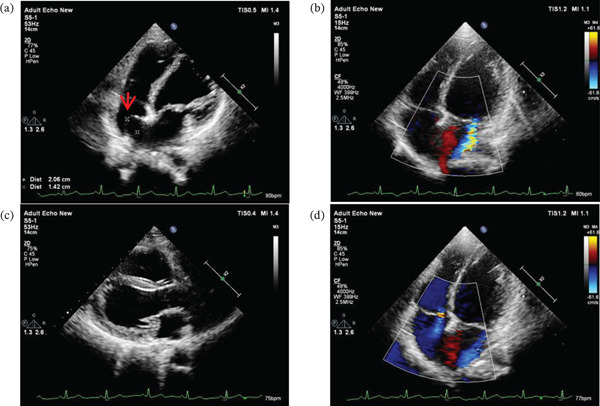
Echocardiogram of mother of proband. (a) Atrial septal aneurysm. (b) Thickened mitral valve with regurgitation. (c) Mitral valve prolapse. (d) Tricuspid regurgitation.
